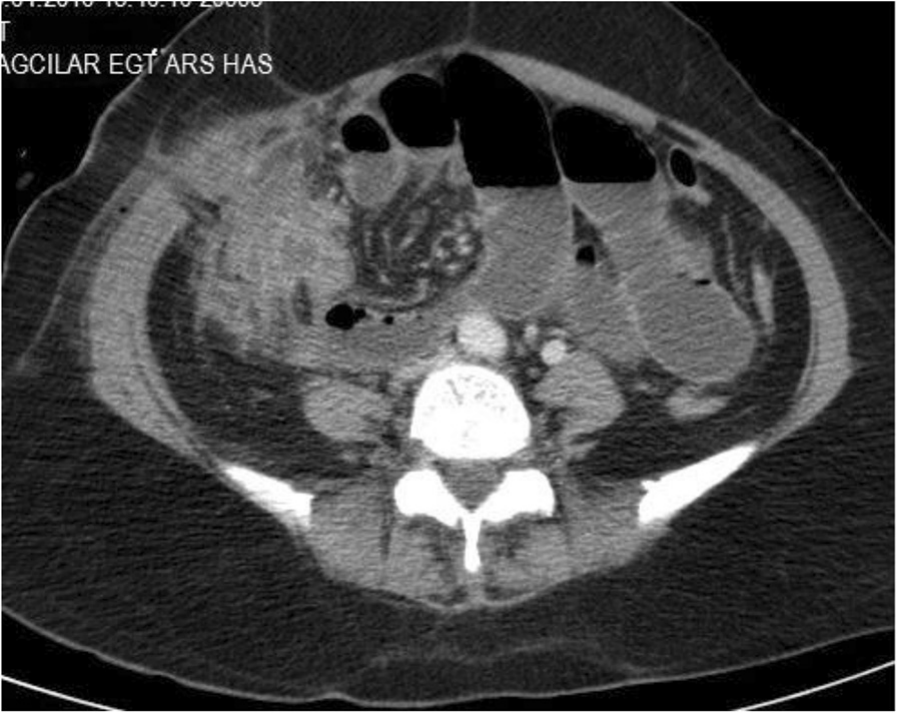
Small bowel and cecal tuberculosis: Contrast-enhanced CT scan shows wall thickening in several distal small bowel loops and cecum.
